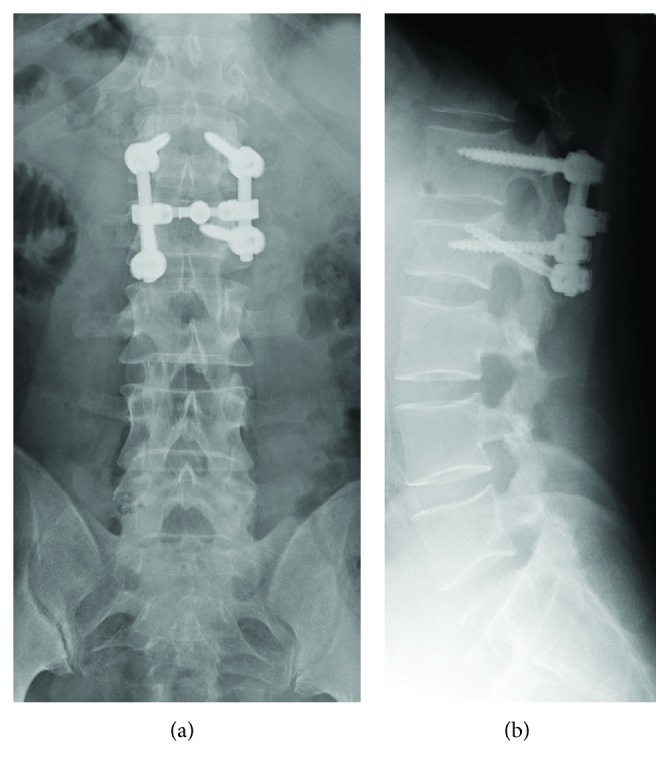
Postoperative plain X-rays of the lumbar spine. (a) Anteroposterior and (b) lateral radiographs show an L1-L2 single-level instrumented fusion using a CBT pedicle screw for L2 on the left side.
